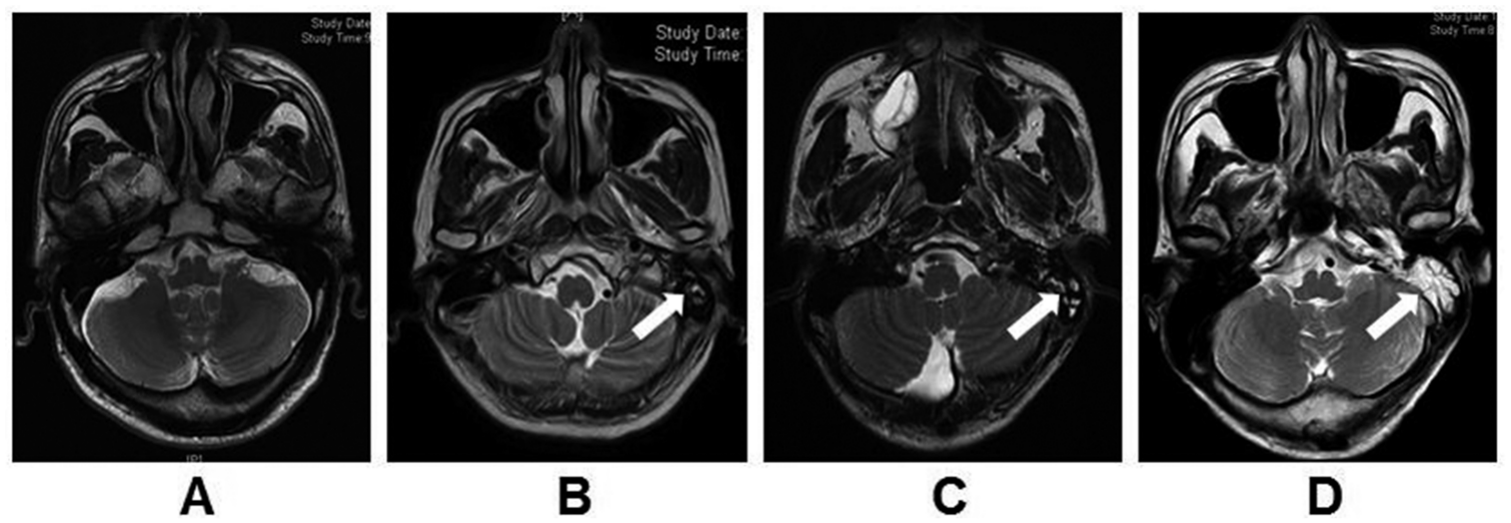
Representative T2 weighted MR images of different grades of mastoiditis. (A) Grade 0 mastoiditis, < 5% of the mastoid air cell is opacified; (B) Grade 1 mastoiditis, 6–33% of the mastoid air cell is opacified; (C) Grade 2 mastoiditis, 34–67% of the mastoid air cell is opacified; (D) Grade 3 mastoiditis, 68–100% of the mastoid air cell is opacified. A high-signal intensity area (white arrow) is observed in mastoid air cells in the left ear; the mastoid air cells in the right ear appear normal.
